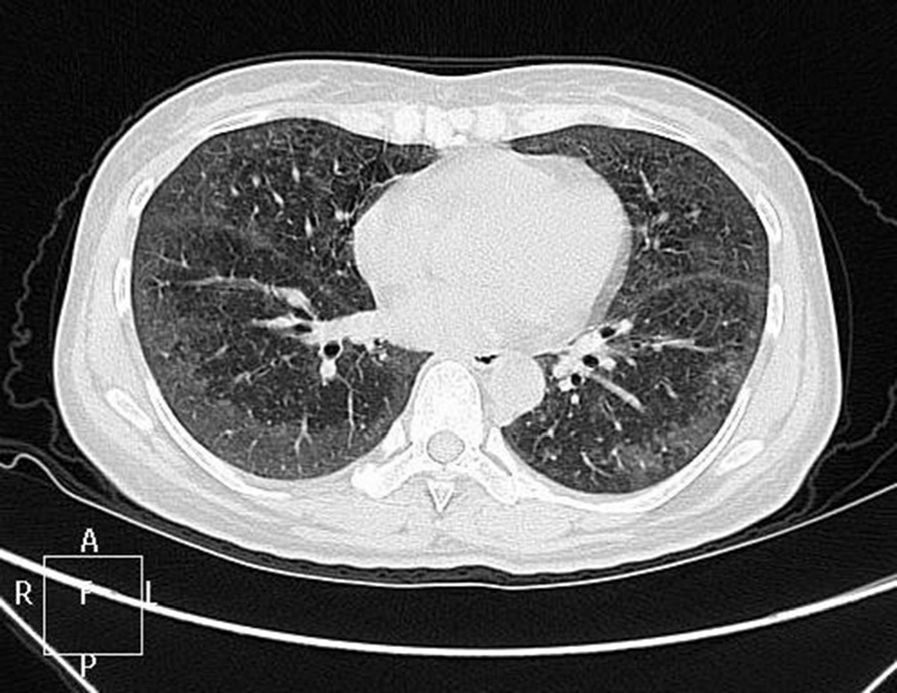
chest CT-scan showed diffused interstitial shadows.
